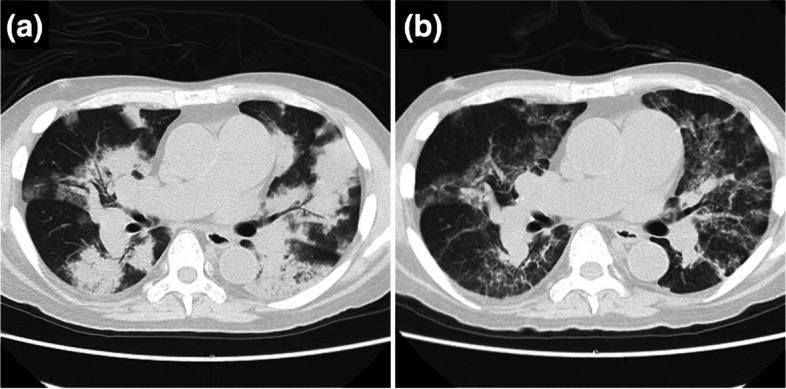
Chest computed tomography images. Computed tomography images showed bilateral diffuse ground-glass opacities and infiltrates upon admission (a). After 15 days, the ground-glass opacities and infiltrates showed improvement (b)
